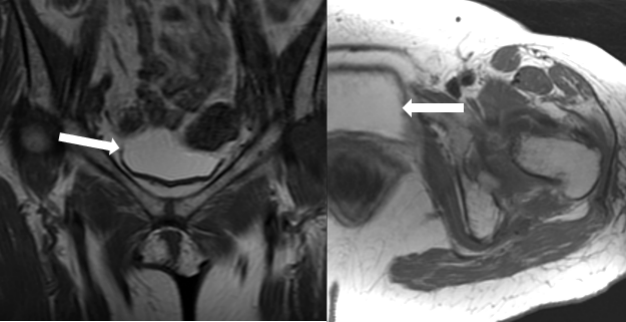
71-year-old female with metastatic pulmonary adenocarcinoma whopresented with lower back pain and underwent gadolinium-enhanced MRI of spine(not shown). Two days later, MRI of pelvis was performed. (a) Coronal and (b)axial precontrast T1W images demonstrate high T1W-SI in bladder urine (whitearrows), equal in SI to that of nearby fat.
